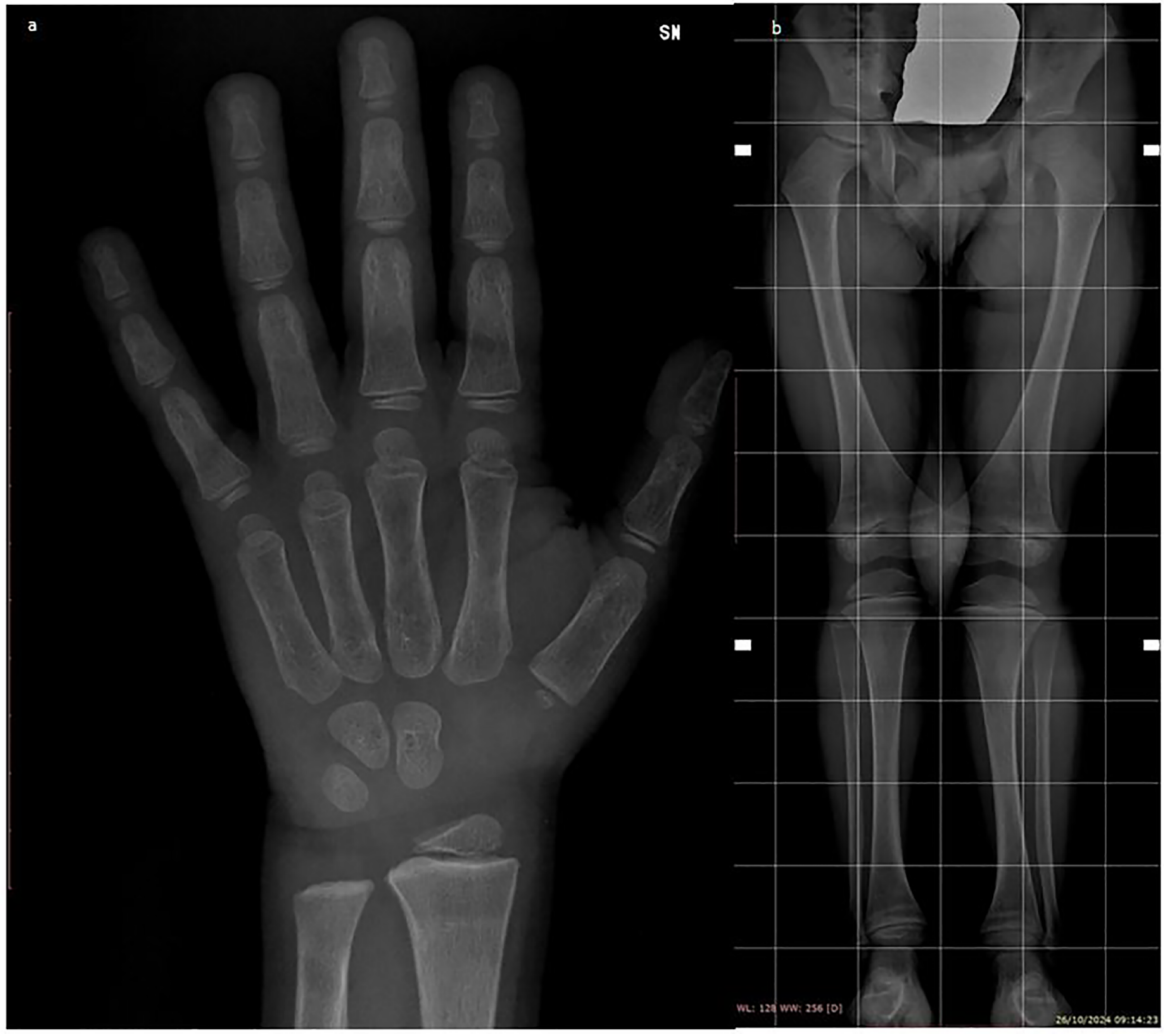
Radiographic improvements in rachitic changes of the wrist (a) and knees (b) after six months of treatment.
